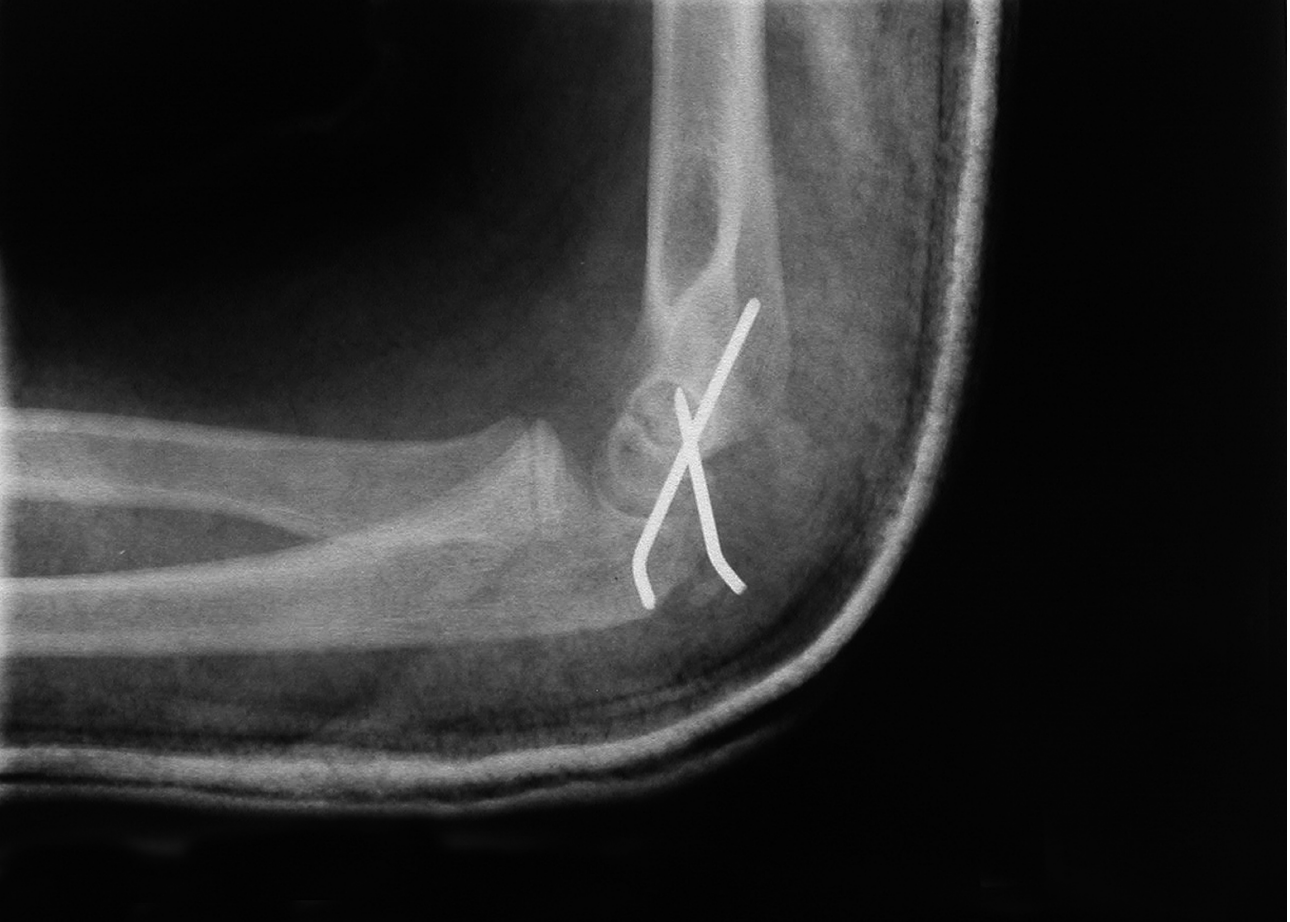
Postoperative lateral radiograph of the elbow revealing internal fixation of the lateral condyle fracture with the help of 2 K-wires.
